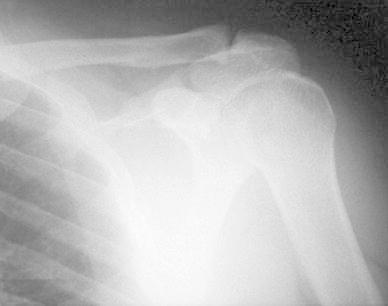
Preoperative roentgenogram, first intervention
